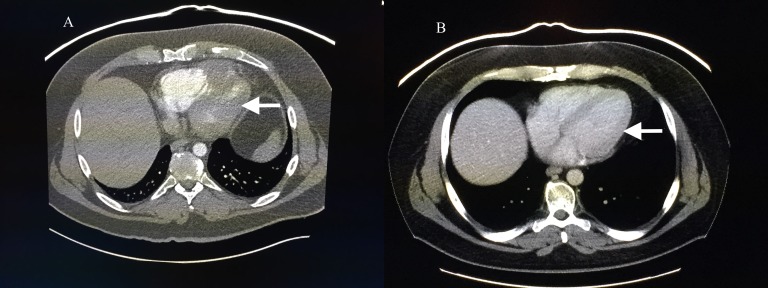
Response to Nivolumab CT scan of the chest showing extent of cardiac involvement (A) before and (B) after nivolumab therapy.
